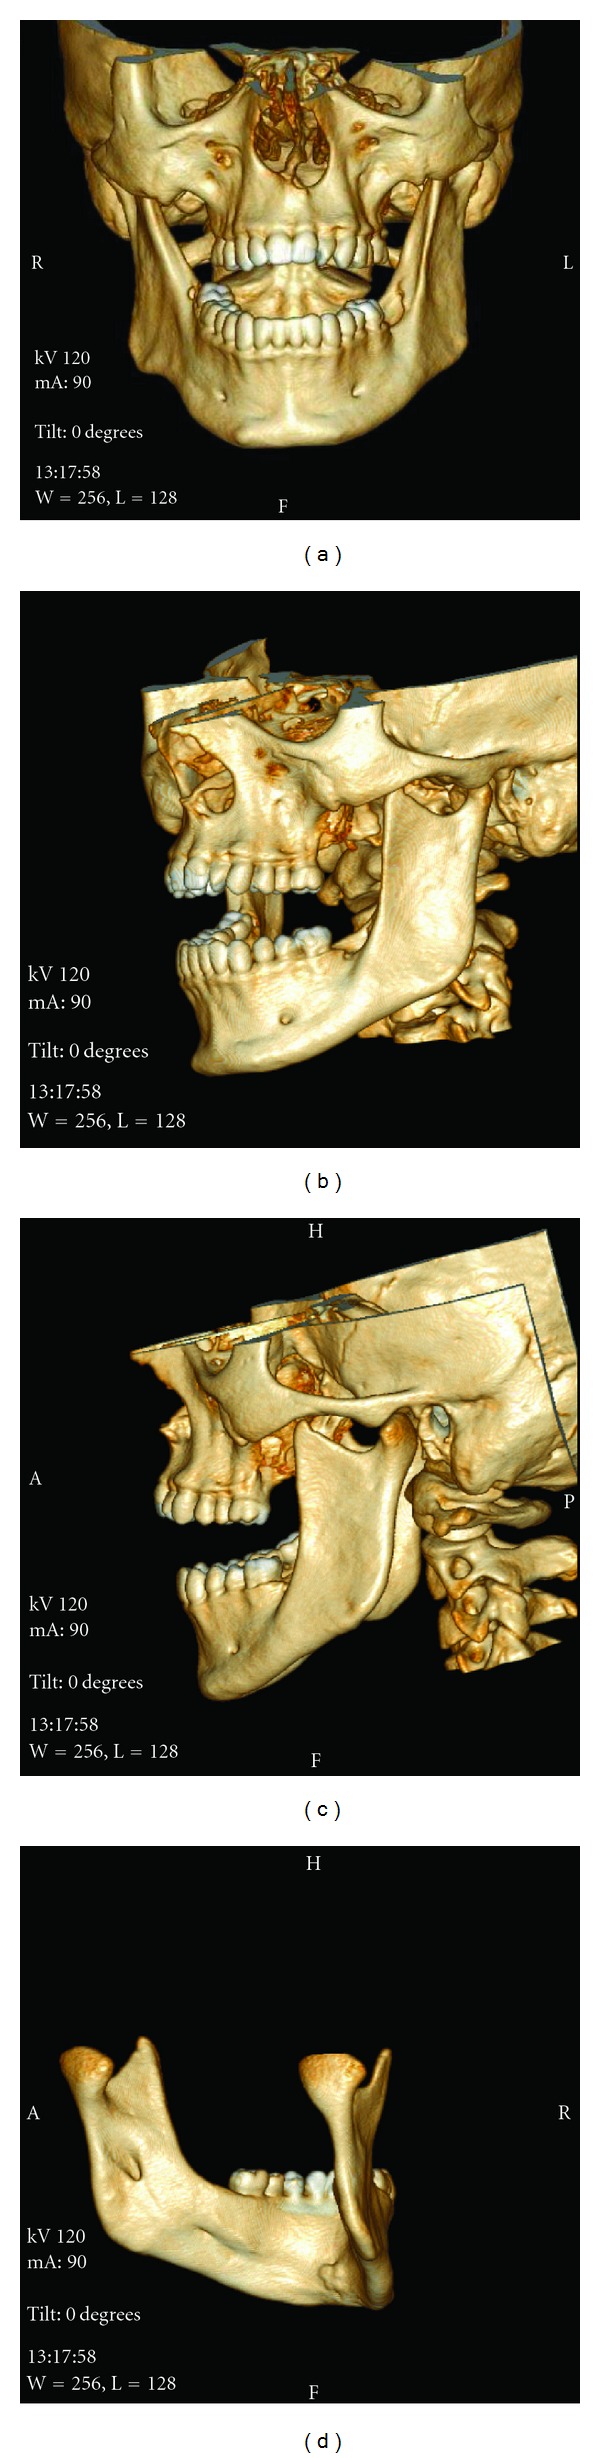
3D CT frontal (a) sagittal (b,c) and lingual (d) images demonstrate the normal bony structure of mandible in all direction after 3 year follow up.
